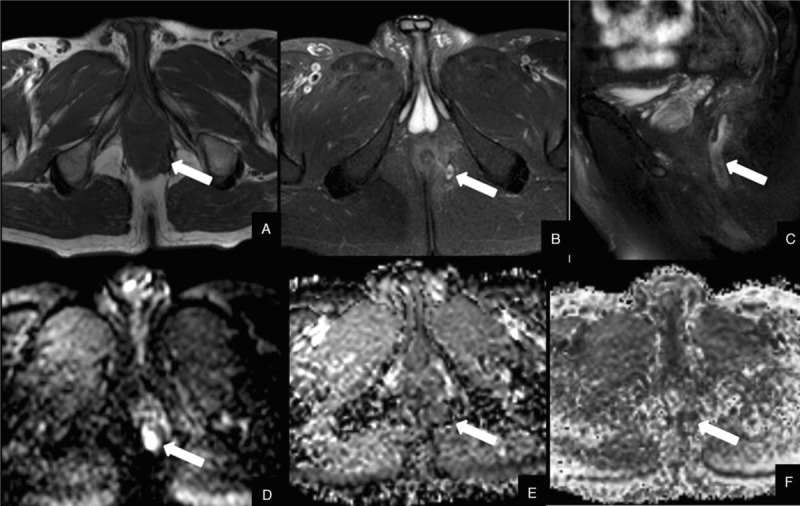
A 34-year-old man with transsphinteric anal fistula of positive inflammation activity with abscess. (A) Axial T2-weighted image shows a perianal fistula (arrow) that exhibits slightly high signal intensity. (B and C) Axial and sagittal fat-suppression T2-weighted images show a perianal fistula (arrow) that exhibits high signal intensity. (D) Axial diffusion tensor imaging reveals high signal intensity at the fistula (arrow). (E) Axial apparent diffusion coefficient (ADC) map shows a value of 0.377 × 10^−3^ mm^2^/s for the fistula. (F) Axial fractional anisotropy (FA) map shows a value of 0.073.
